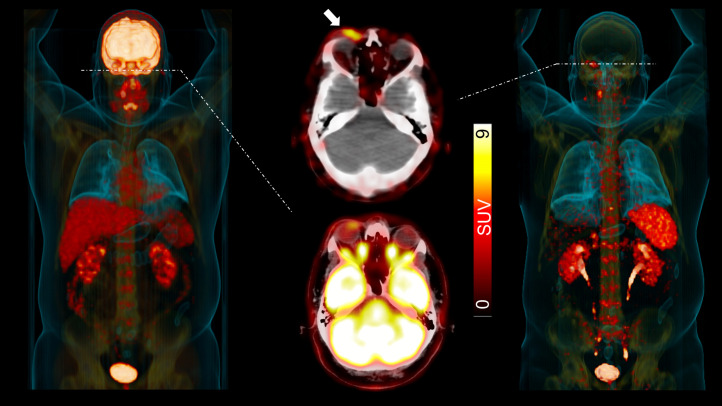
MZL patient with additional periorbital disease site (white arrow) identified on ^68^Ga-pentixafor PET/CT. On ^18^F-FDG maximum-intensity projection (MIP, left) and transaxial PET/CT (middle, bottom), periorbital manifestation was masked by normal biodistribution in brain. On CXCR4-targeted ^68^Ga-pentixafor (MIP, right; transaxial PET/CT, middle top), this additional site of disease can be identified because of missing brain accumulation. (Modified from (53).)
